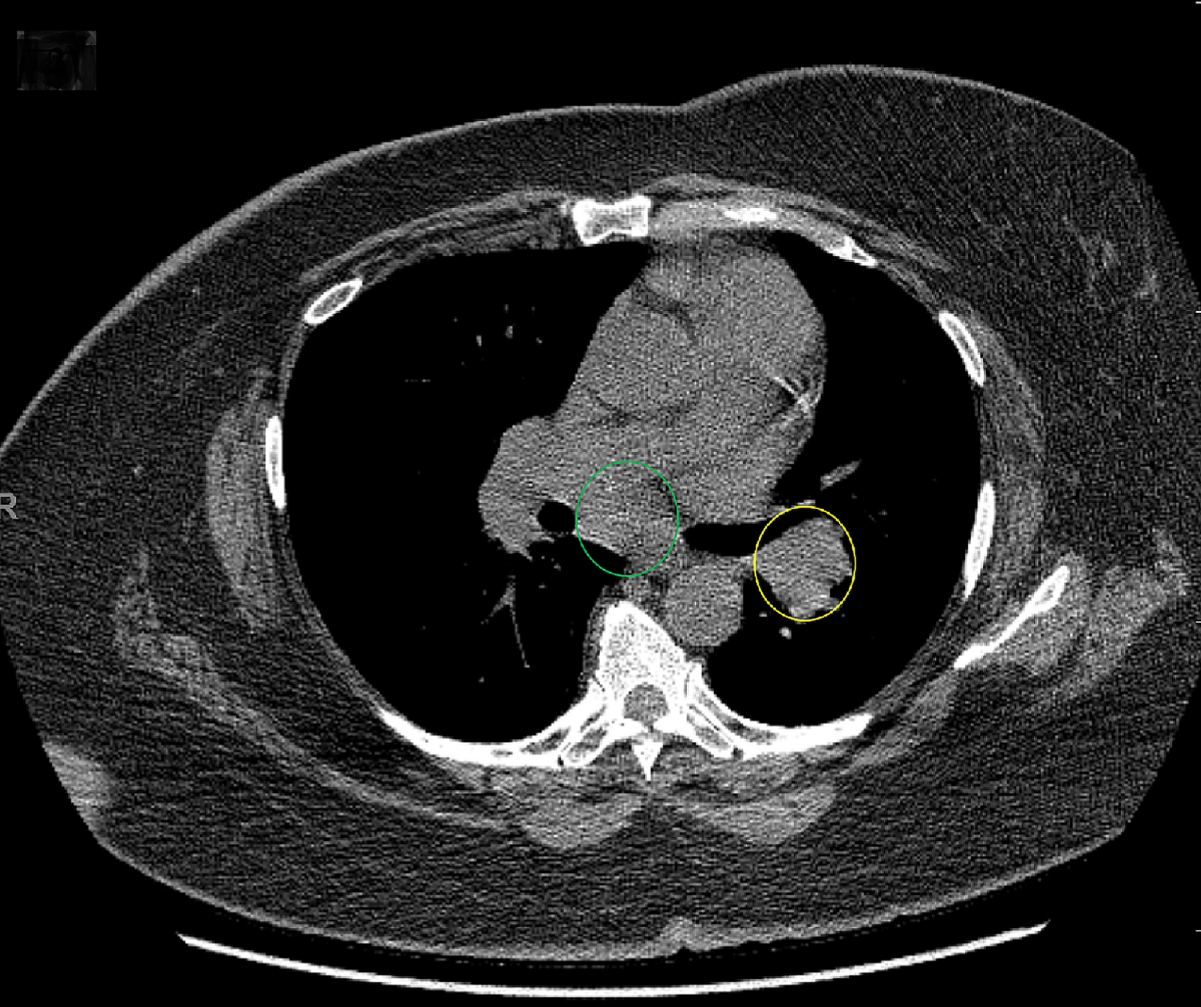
Axial Computed Tomography Scan of the Chest in Soft Tissue Window Prominent hilar (yellow circle) and mediastinal (green circle) lymphadenopathy is seen.
